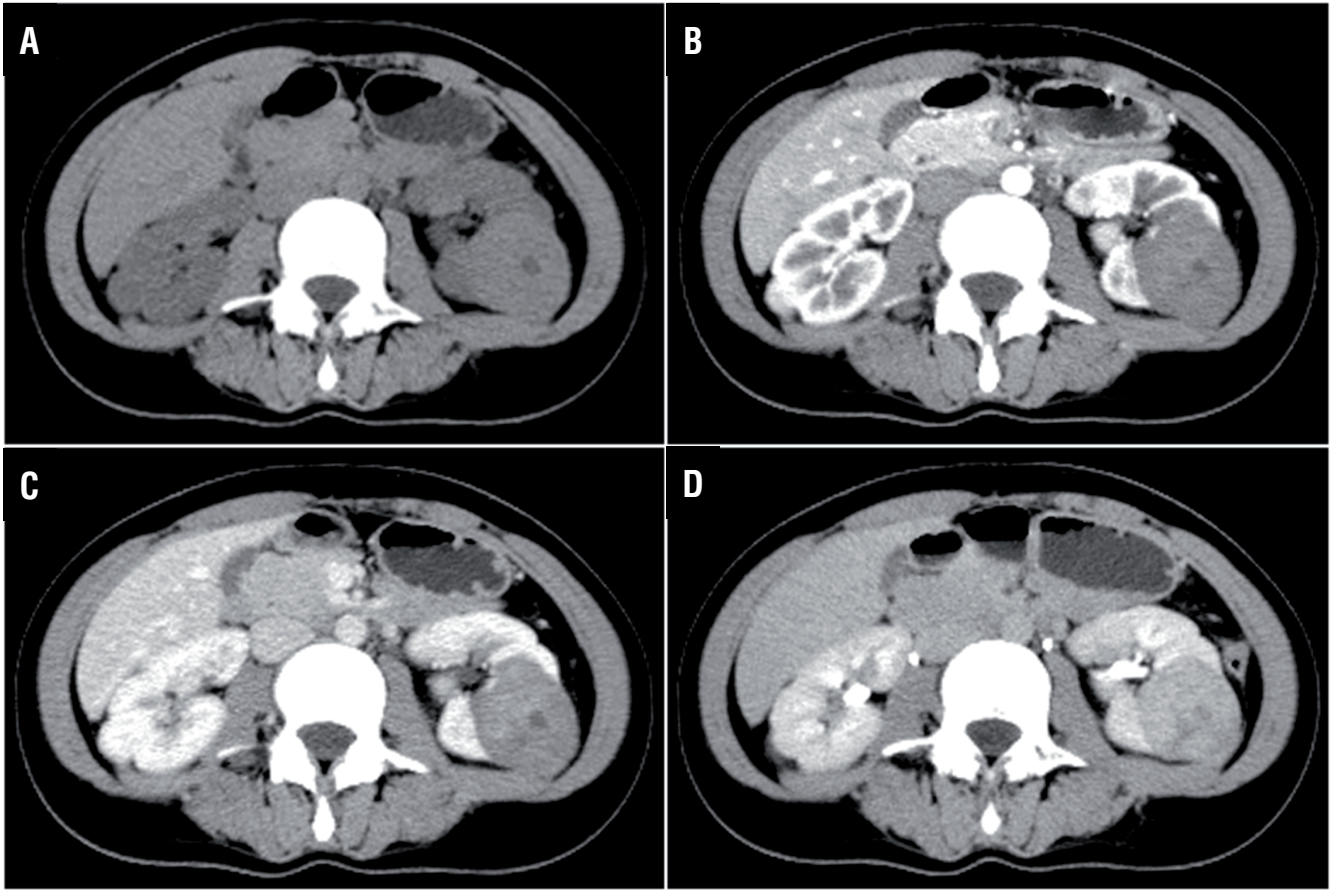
Enhanced computed tomography scan (CT) demonstrated a 4.5×4.0×4.5cm neoplasm in the middle and dorsal part of left kidney (CT unit: 45). It had a clear boundary and part of the neoplasm extruded the renal contour. Multiple patchy low density area could be seen in the neoplasm (CT unit: 10). The neoplasm was lightly enhanced in the arterial phase (CT unit: 57.2), and it was continuously enhanced in the venous (CT unit: 68.9) and excretory phase (CT unit: 88.8). The low density area did not show any enhancement.
